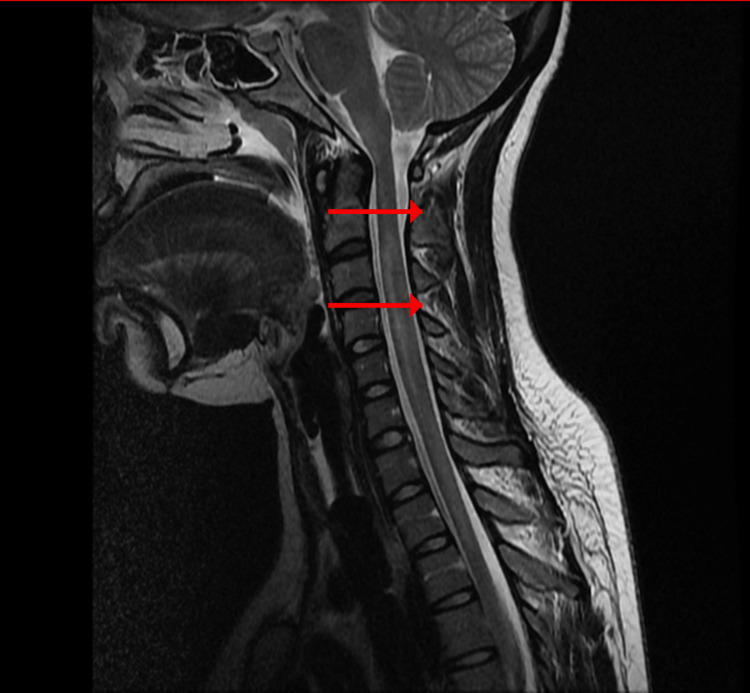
Sagittal T2-weighted cervical spinal MRI showing longitudinal extensive transverse myelitis Sagittal T2-weighted image demonstrating a continuous intramedullary hyperintense lesion extending from C2 to C6 (arrows). MRI: Magnetic resonance imaging.
